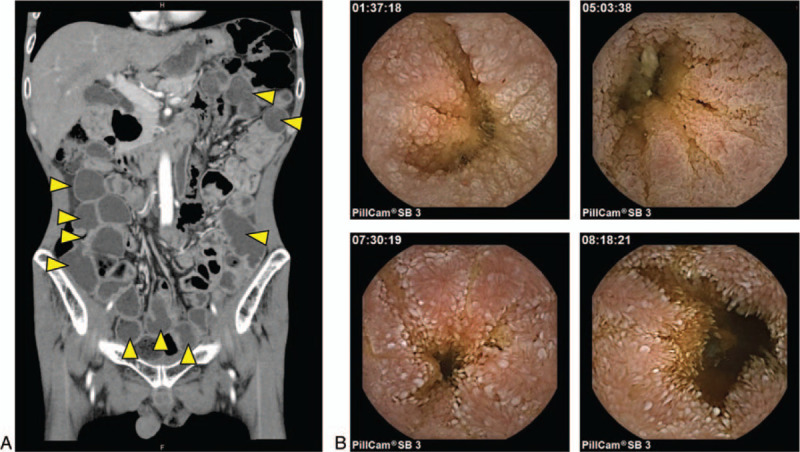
(A) Contrast-enhanced CT on admission. The small intestine contained excessive fluid (Yellow Arrowheads) but showed no obvious wall thickness. (B-E) Capsule endoscopy showed unique images with white villi in the entire small intestine. Small bowel transit time was 8 hour 21 minutes.
